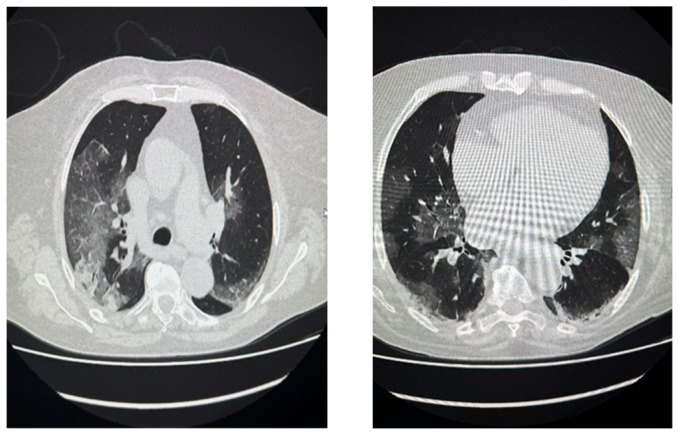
High-resolution computed tomography (HR-CT) of the chest. In both lungs, patchy areas of interstitial opacities of ground-glass appearance, with features of consolidation at the base of the right lung. In both lungs, scar-like fibrotic changes with thickening of the interlobular septa and features of bronchial traction. The appearance is typical in the course of COVID-19 infection (patient: 68-year-old female, day 9 of SARS-CoV-2 infection).
